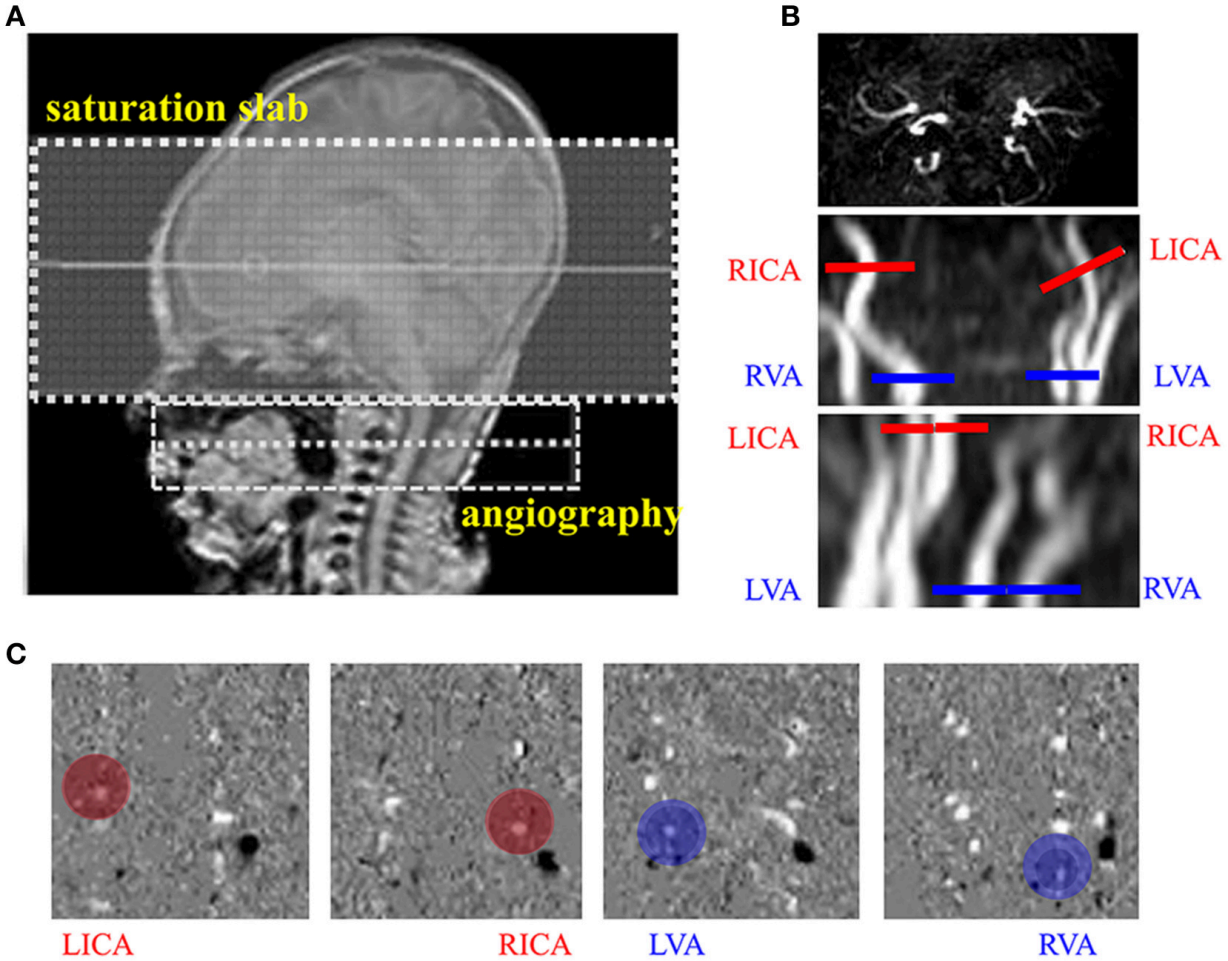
Measurement of cerebral blood flow (CBF) using phase contrast (PC) MRI. (A) Positioning of magnetic resonance angiography (MRA). (B) Positioning of PC MRI for the left internal carotid arteries (LICA), right internal carotid arteries (RICA), leftvertebral arteries (LVA) and right vertebral arteries (RVA) using the maximal-intensity-projection (MIP) images of the TOF angiogram. (C) Example velocity maps of the LICA, RICA, LVA, and RVA. Blood flow velocity was measured in the velocity map (red and blue circle).
